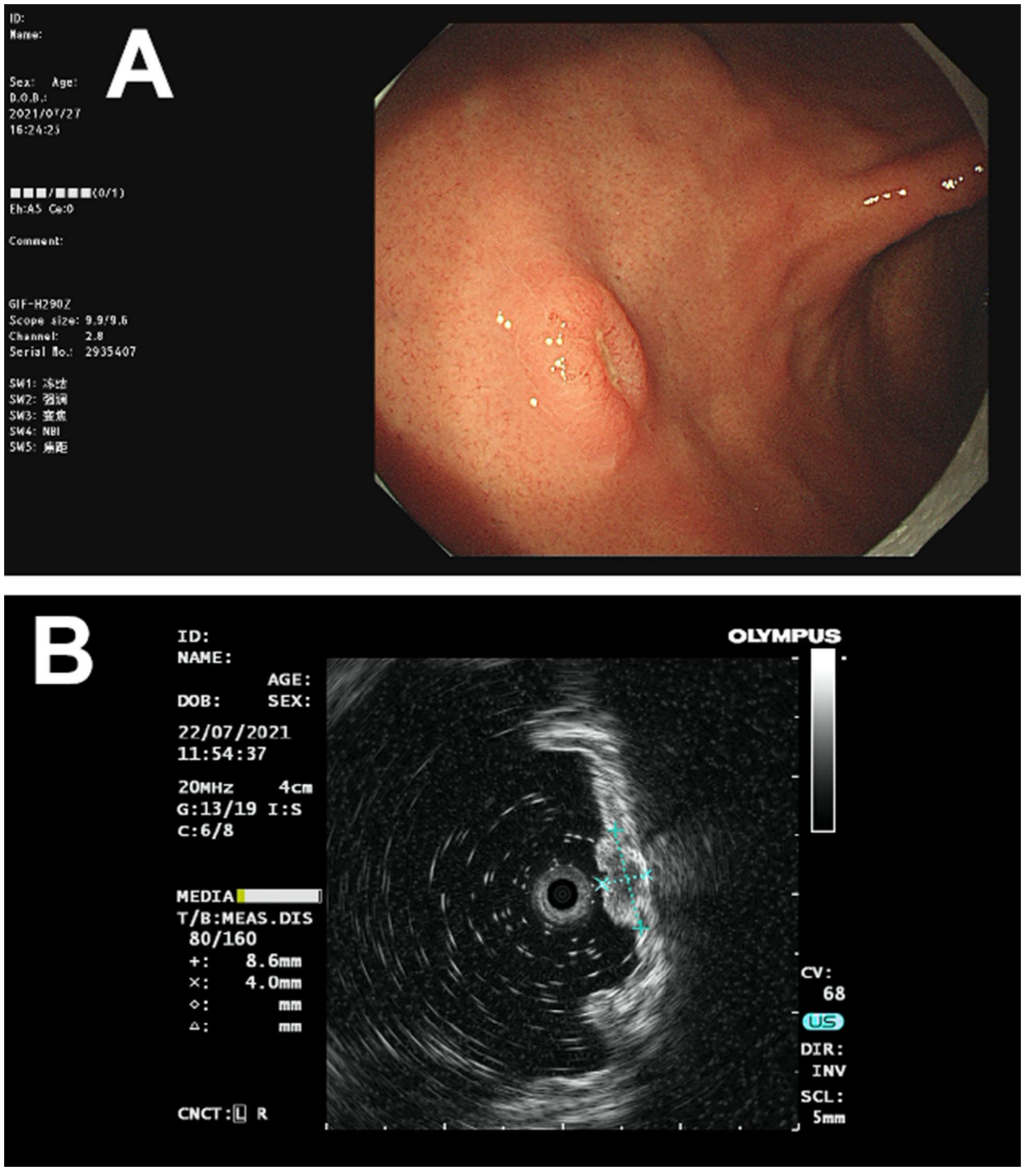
The images of gastric lesions obtained through endoscopy and endoscopic ultrasound. (A) Gastroscopy revealed a broad-based raised lesion, approximately 1.0 cm in diameter, on the anterior wall of the gastric body, with a central star-shaped depression, erosion, and surrounding congestion. (B) Endoscopic ultrasonography showed a lesion on the lower anterior wall of the gastric body, invading the submucosal layer.
